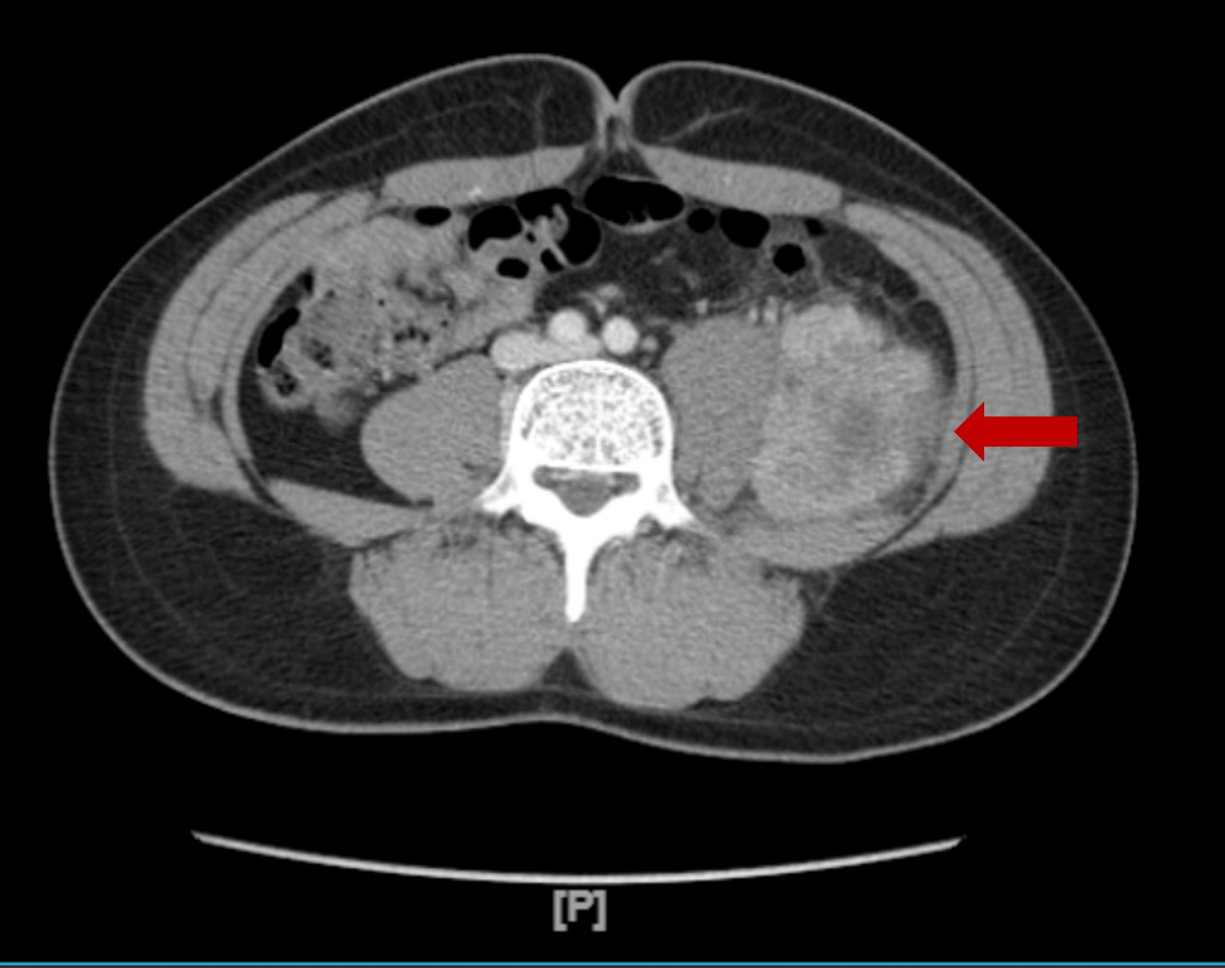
Abdominal CT scan with IV contrast showing retroperitoneal Ewing sarcoma (red arrow) CT: computed tomography; IV: intravenous
